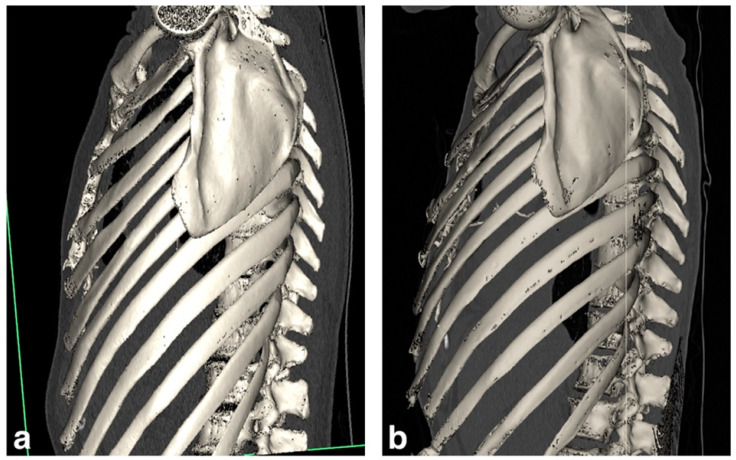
Patient Nº3. 3D reconstructions obtained from CT scans pre- (a) and 18 months post- (b) lung transplantation highlight the reversion of the flat chest of the patient.
